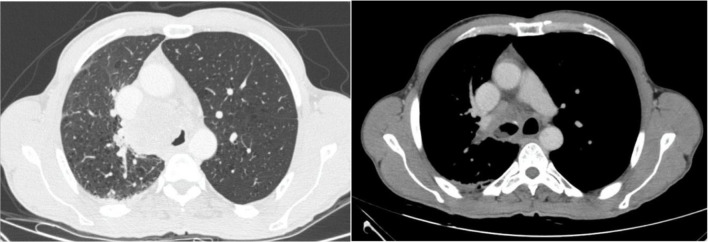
Chest computed tomography scans showing a large mass in the right upper lung lobe with tracheal and mediastinal infiltration.
